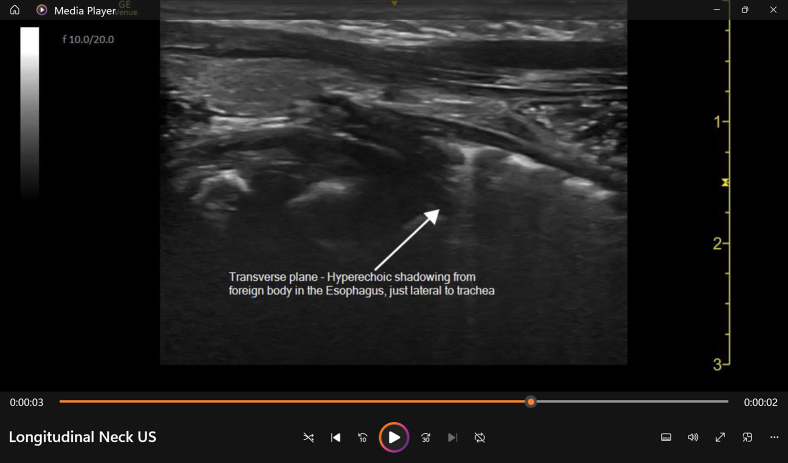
Longitudinal neck ultrasound showing posterior acoustic shadowing within the esophagus, noted by the arrow. The trachea is seen without posterior acoustic shadowing.
